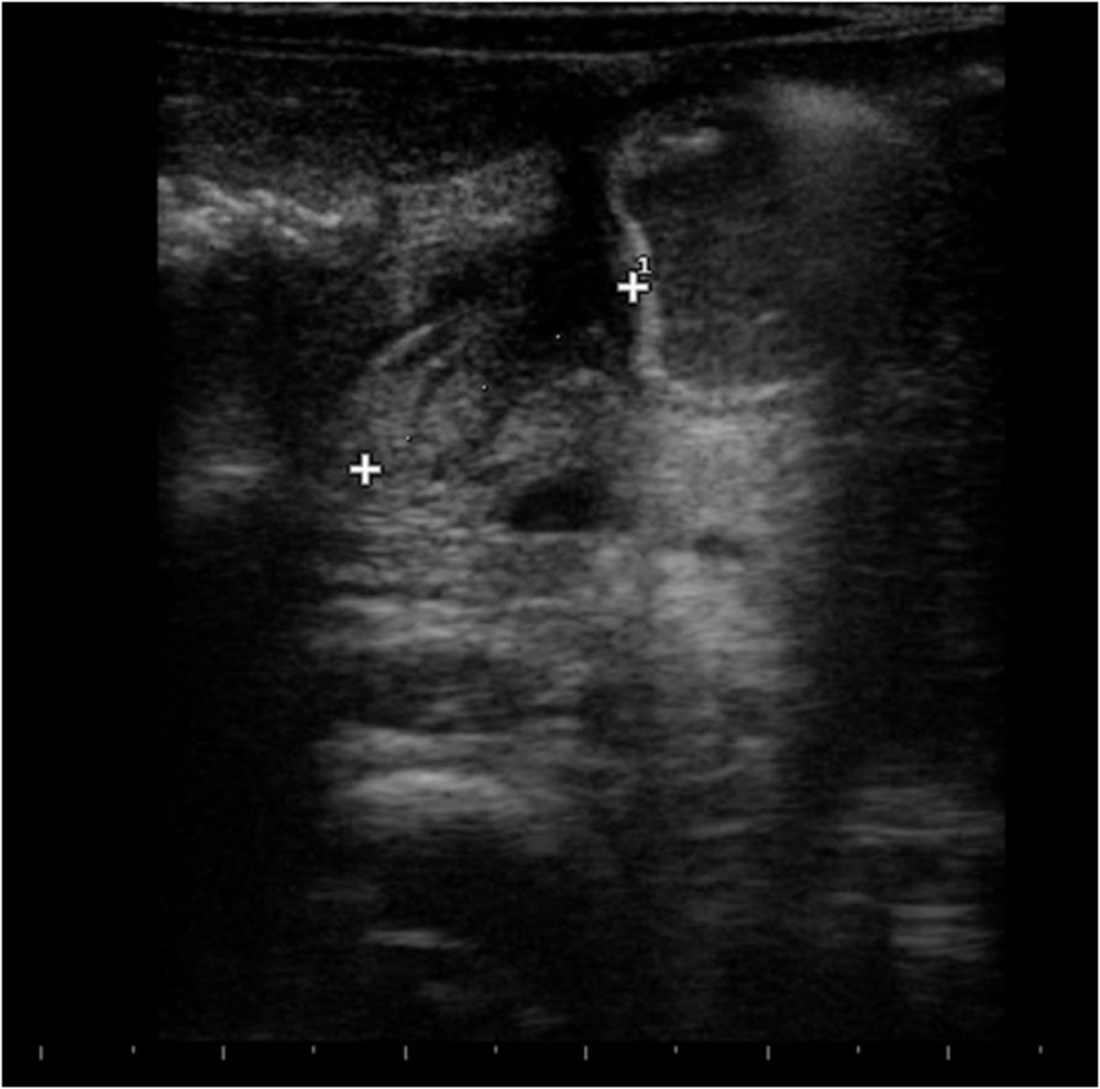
Ultrasound features of hypertrophic pyloric stenosis. On this view, we evaluate the “double track sign” characterized by double layer of redundant mucosa hiper-echoic separated by several linear tracts of contrast material, that occludes the lumen of the stomach protrudes into the liquid and antral .On this scan the length of pyloric canal and in our experience is >1.3cm mean 1.5cm.
